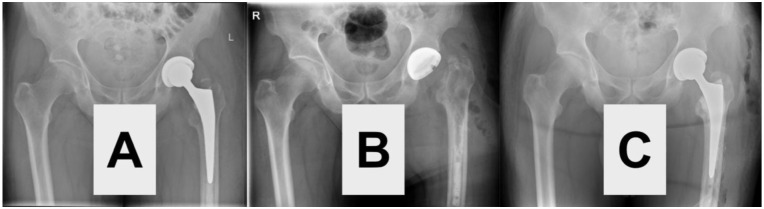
Radiographic findings of a pseudoaneurysmal bone cyst in the setting of primary hyperparathyroidism. (a) Pelvic radiograph shows a total hip arthroplasty on the left side preoperatively, while the left femoral head exhibits a multiloculated, expansile osteolytic lesion with cortical thinning and septation, radiographically consistent with a brown tumor or pseudoaneurysmal bone cyst. (b) Anteroposterior view of the pelvis without prosthesis, demonstrating the same osteolytic lesion of the proximal right femur with ballooning of the cortex and well-defined margins, suggesting a benign but aggressive lesion. (c) Follow-up radiograph post-treatment (after parathyroidectomy and correction of hyperparathyroidism) shows reossification and partial consolidation of the cystic bone lesion, consistent with healing of a brown tumor. These findings are consistent with a brown tumor (osteitis fibrosa cystica), a reactive bone lesion secondary to excess PTH in primary hyperparathyroidism. The lesion’s pseudoaneurysmal appearance can mimic neoplastic or vascular pathology but is reversible upon correction of the underlying endocrine disorder. PTH: parathyroid hormone.
